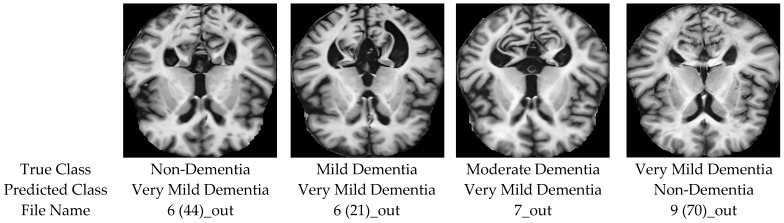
Misclassified MRI slices from the EfficientNet + Swin Transformer ensemble.
